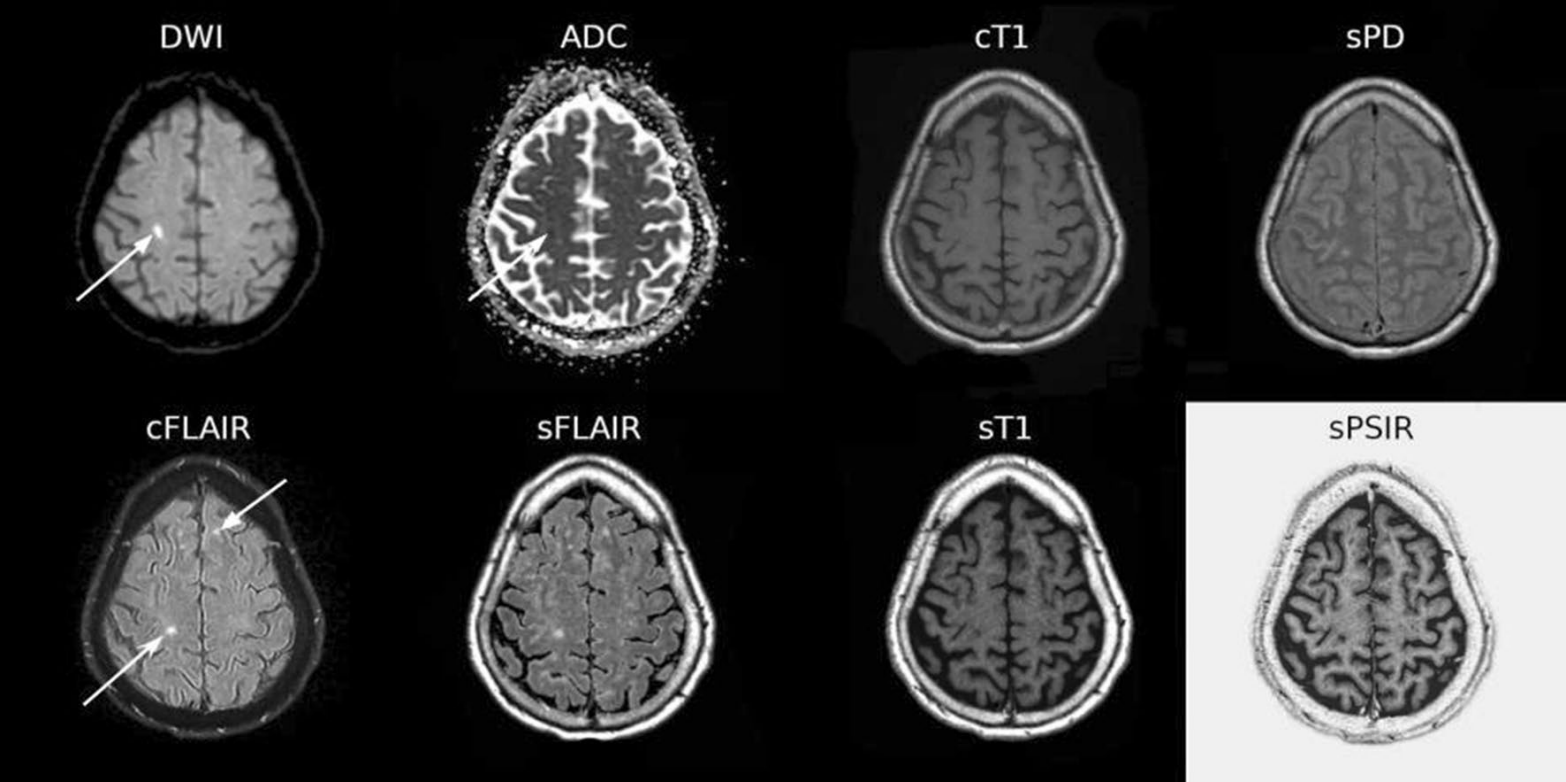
Axial conventional (DWI, ADC, cFLAIR, cT1) and synthetic (sFLAIR, sT1, sPD, sPSIR) weighted images depicting acute (DWI + /rADC, arrowhead) and chronic (WMH, arrow) ischemic lesions.
